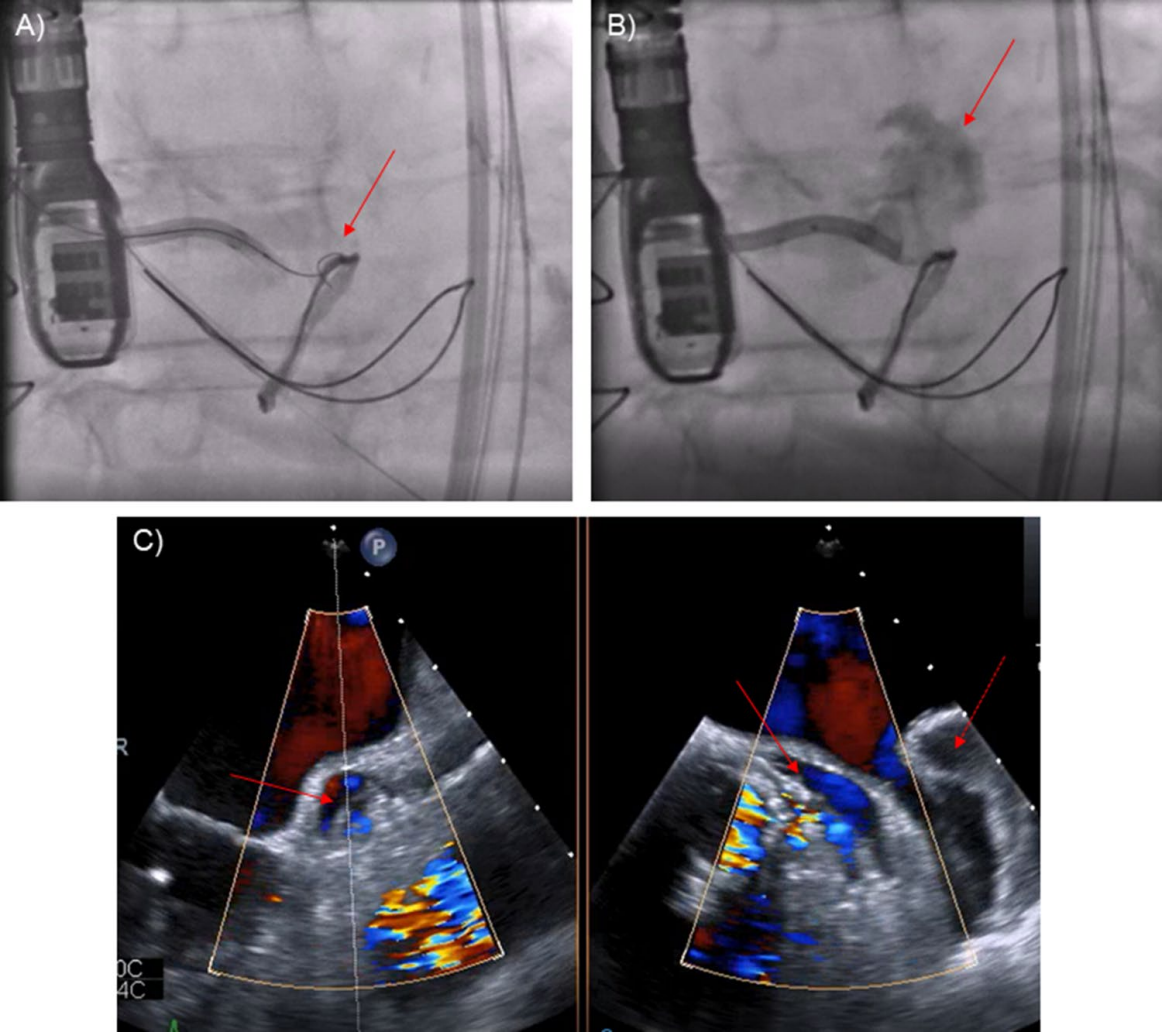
BASILICA procedure I. Traversal attempt with deviation of the electrified wire into the Sinus of Valsalva (a, solid arrow) causing laceration confirmed by angiography (b, solid arrow) and echocardiography (c, solid arrows). Left atrial wall hematoma (c, dotted arrow)
